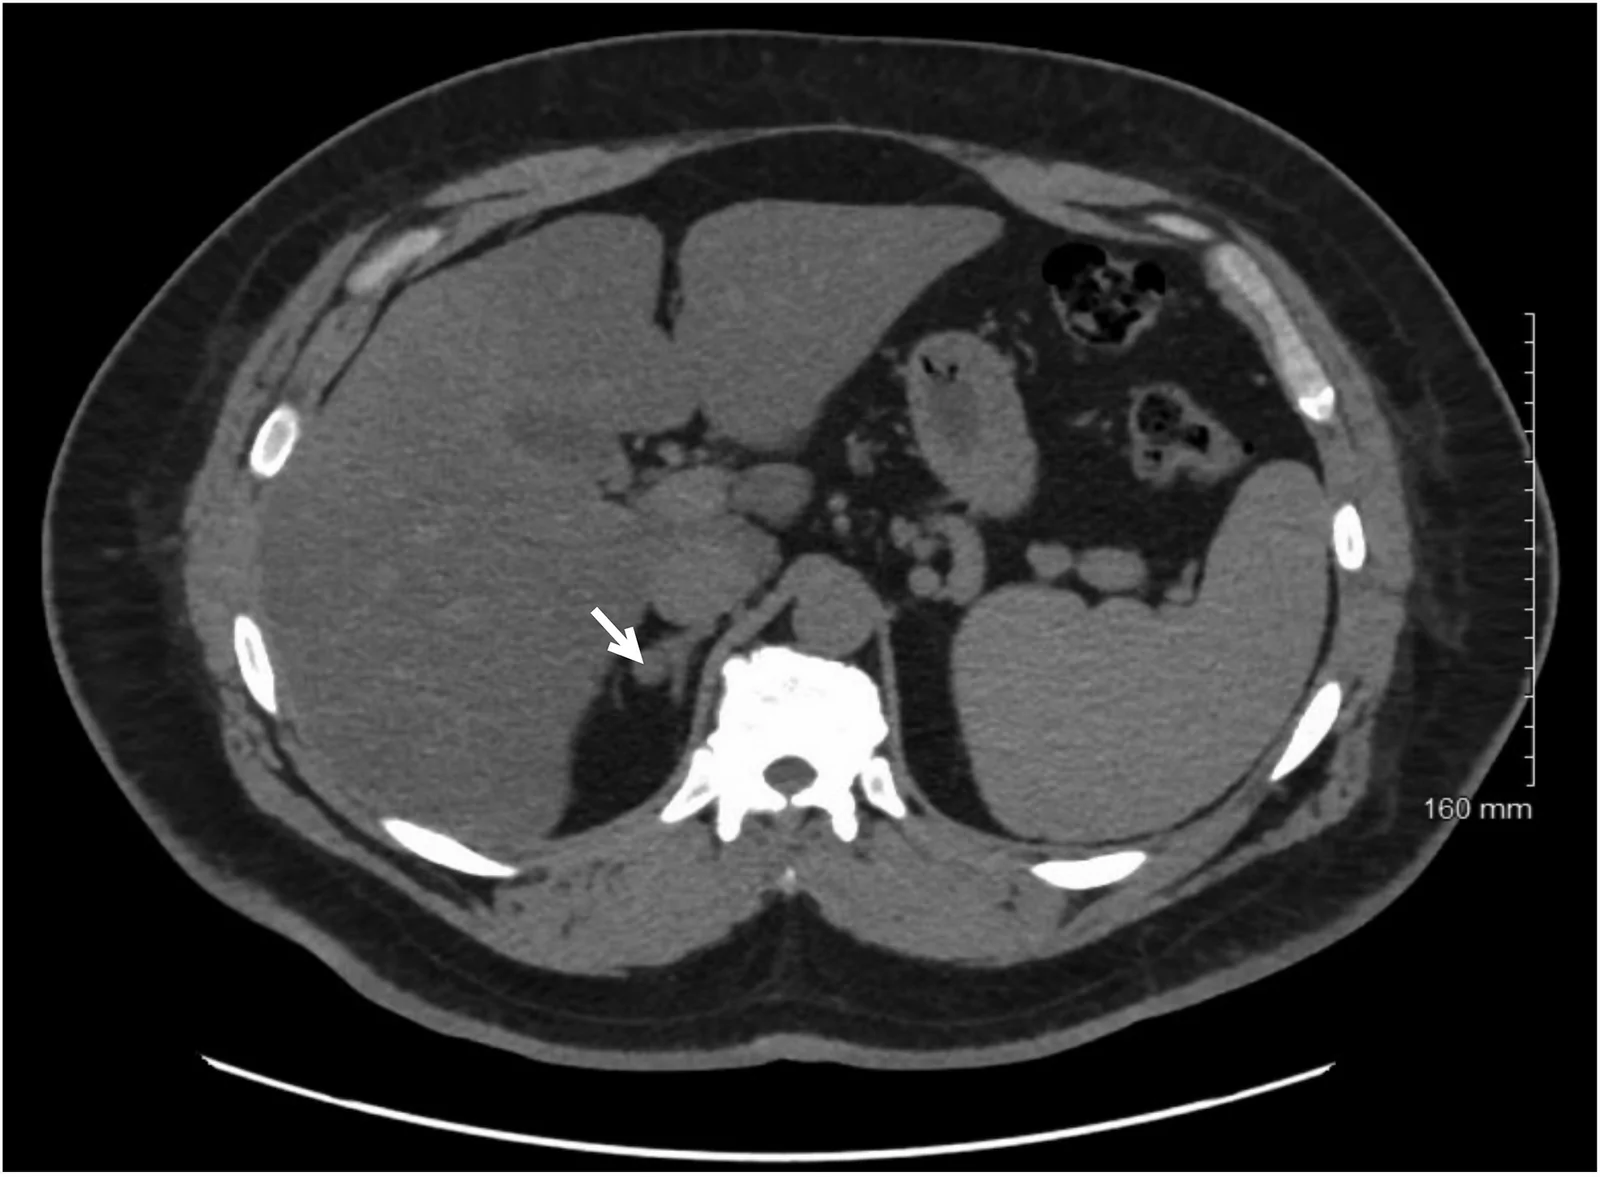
Right adrenal nodule with an unenhanced computed tomography attenuation of 18.4 Hounsfield units.
